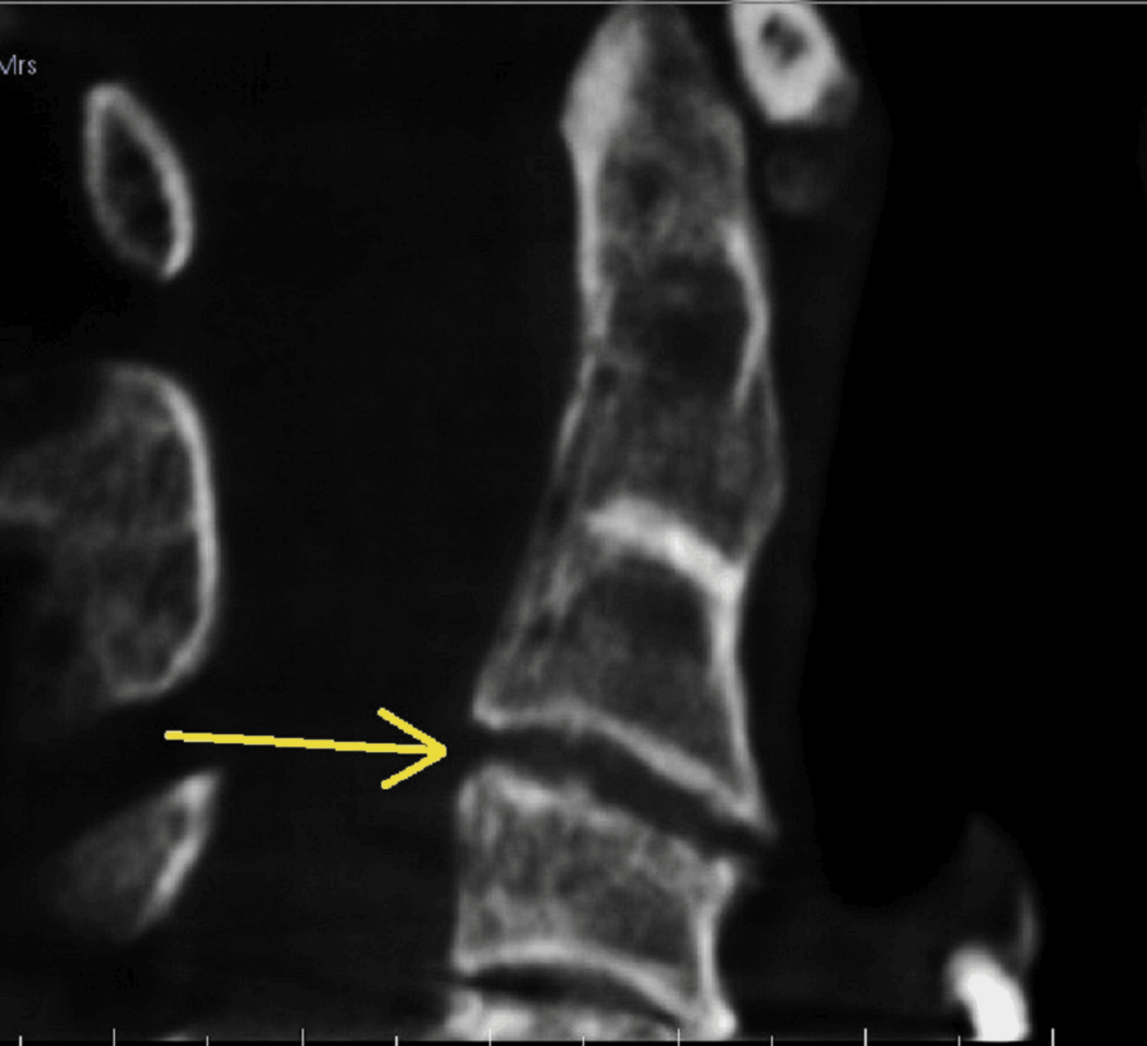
CBCT imaging showing upper cervical vertebrae grade 2. Less than three erosions and small osteophytes. CBCT: cone-beam computed tomography.
